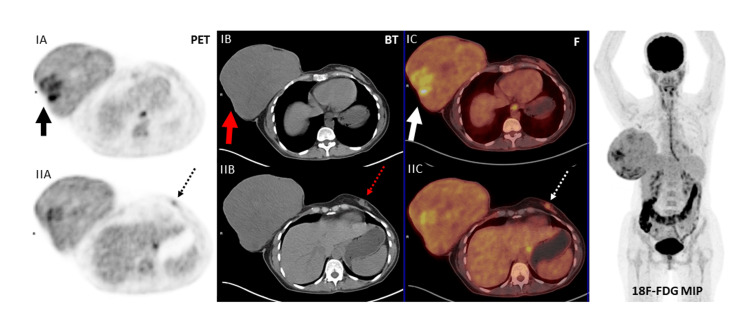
Preoperative PET-CT images of bilateral phyllodes tumor
